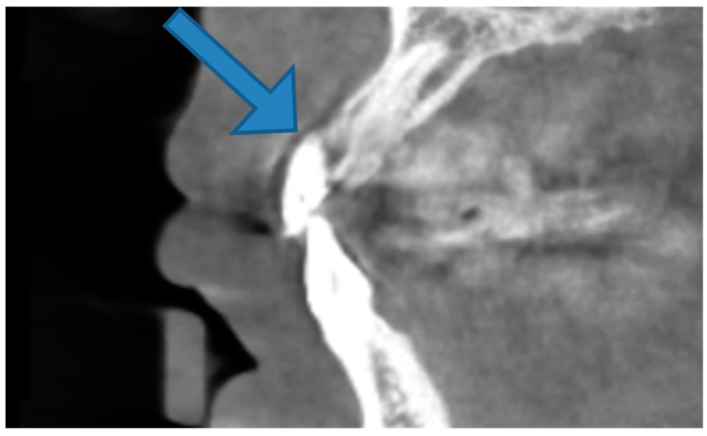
Determination of gingival thickness in CBCT (arrow).
